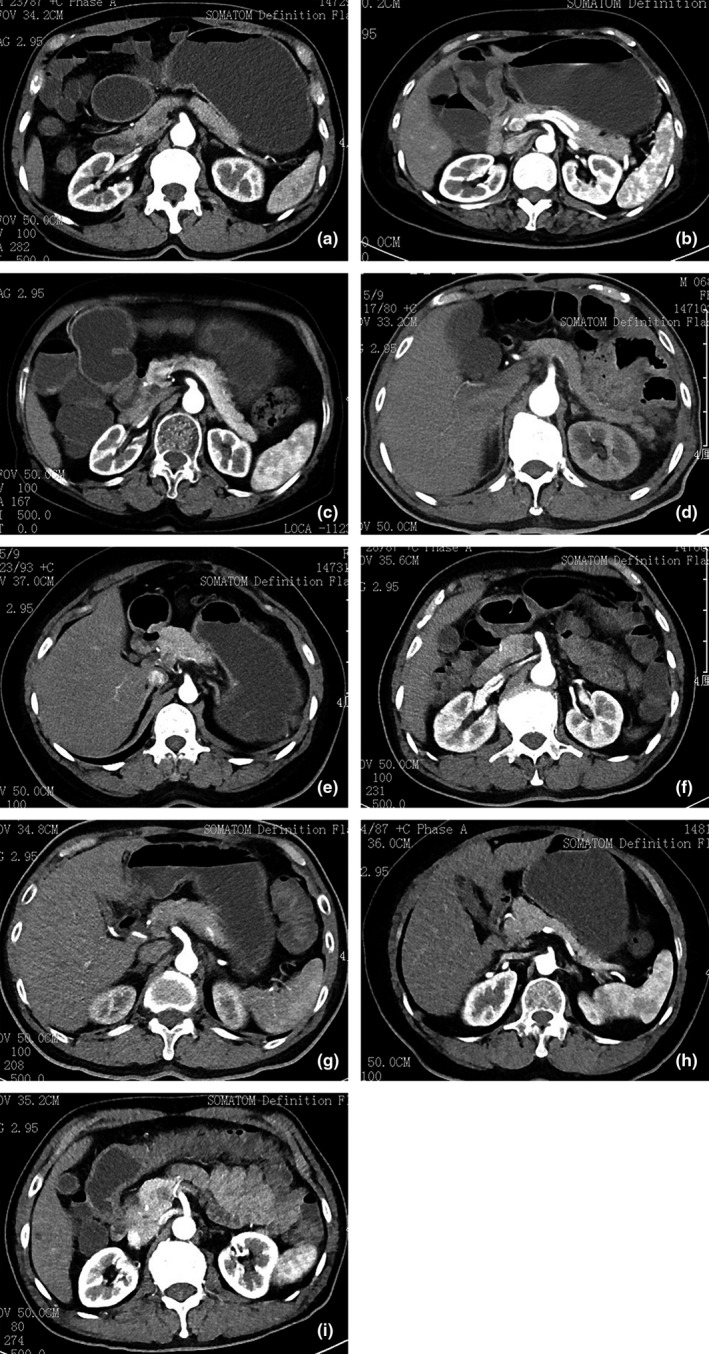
The MSCT radiography of small intestine under different tube voltage and current. (a). The images was reconstructed using FBP under the tube voltage of 120 kV and tube current of 200 mAs at arterial phase (patient A: male, 54 yr, BMI = 21.6). (b)The images was reconstructed by SAFIRE 4 and collected by CARE Dose 4D under the tube voltage of 100 kV and tube current of 295 mAs at arterial phase (patient B: male, 56 yr BMI = 21.3). (c) The images was reconstructed by SAFIRE 4 and collected by CARE Dose 4D under the tube voltage of 100 kV and tube current of 262 mAs at arterial phase (patient C: male, 54 yr, BMI = 21.4). (d) The images was reconstructed by SAFIRE 4 and collected by CARE Dose 4D under the tube voltage of 100 kV and tube current of 230 mAs at arterial phase (patient D: male, 53 yr, BMI = 21.3). (e) The images was reconstructed by SAFIRE 4 and collected by CARE Dose 4D under the tube voltage of 100 kV and tube current of 196 mAs at arterial phase (patient E: male, 55 yr, BMI = 21.7). (f) The images was reconstructed by SAFIRE 4 and collected by CARE Dose 4D under the tube voltage of 100 kV and tube current of 164 mAs at arterial phase (patient F: male, 52 yr, BMI = 21.5). (g) The images was reconstructed by SAFIRE 4 and collected by CARE Dose 4D under the tube voltage of 100 kV and tube current of 131 mAs at arterial phase (patient G: male, 56 yr, BMI = 21.2). (h) The images was reconstructed by SAFIRE 4 and collected by CARE Dose 4D under the tube voltage of 100 kV and tube current of 98 mAs at arterial phase (patient H: male, 53 yr, BMI = 21.3). (i) The images was reconstructed by SAFIRE 4 and collected by CARE Dose 4D under the tube voltage of 80 kV and tube current of 207 mAs at arterial phase (patient I: male, 55 yr, BMI = 21.4). BMI, body mass index
